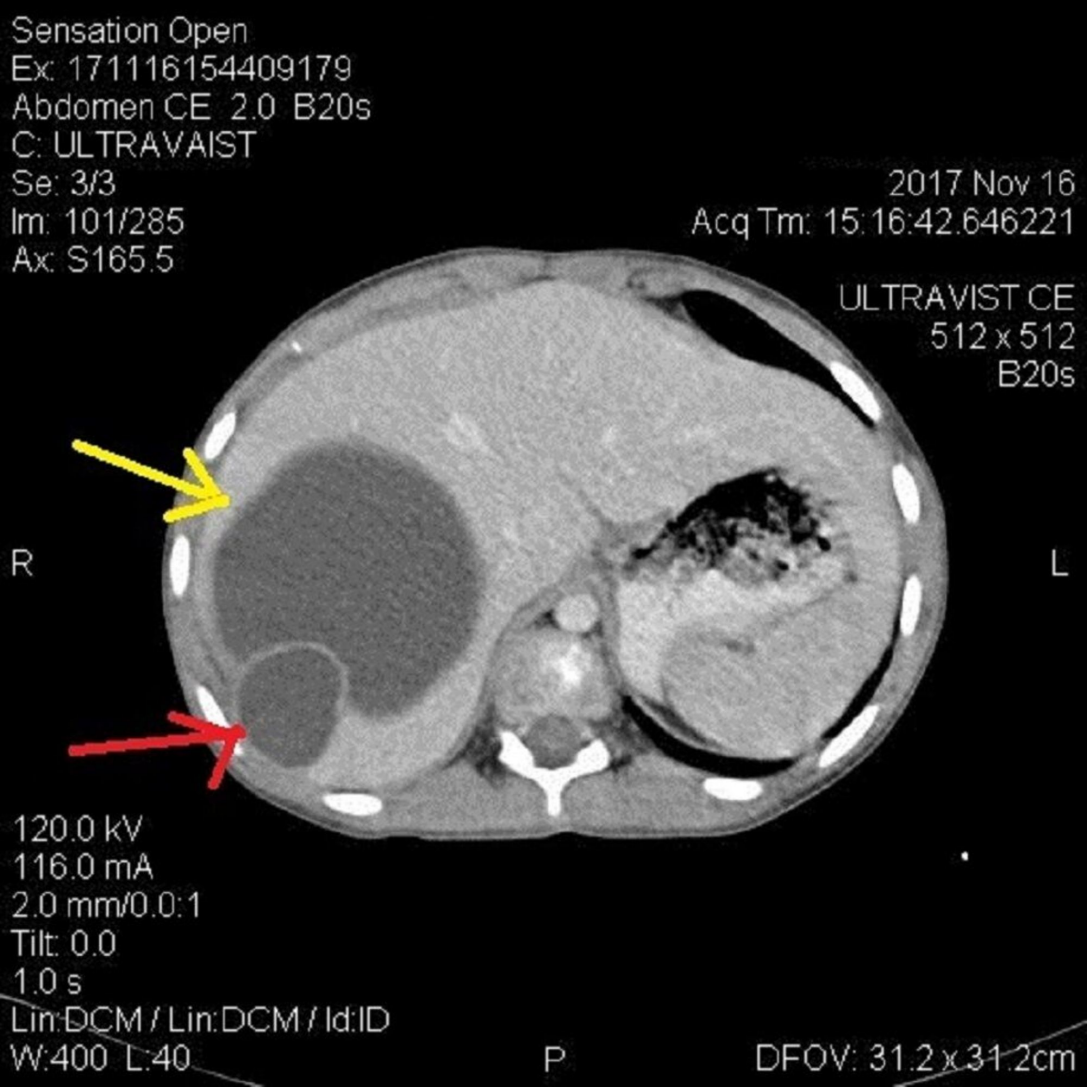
Computed tomography (CT) findings CT abdomen showing multiple cysts in liver.
